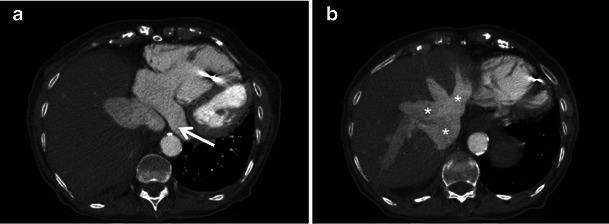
A 75-year-old male with severe tricuspid regurgitation. (a, b) Axial chest CT images demonstrate severe right atrial and ventricular enlargement secondary to severe tricuspid regurgitation. Right heart volume and pressure overload led to severe dilatation of the CS (*arrow*), IVC and hepatic veins (*asterisks*)
